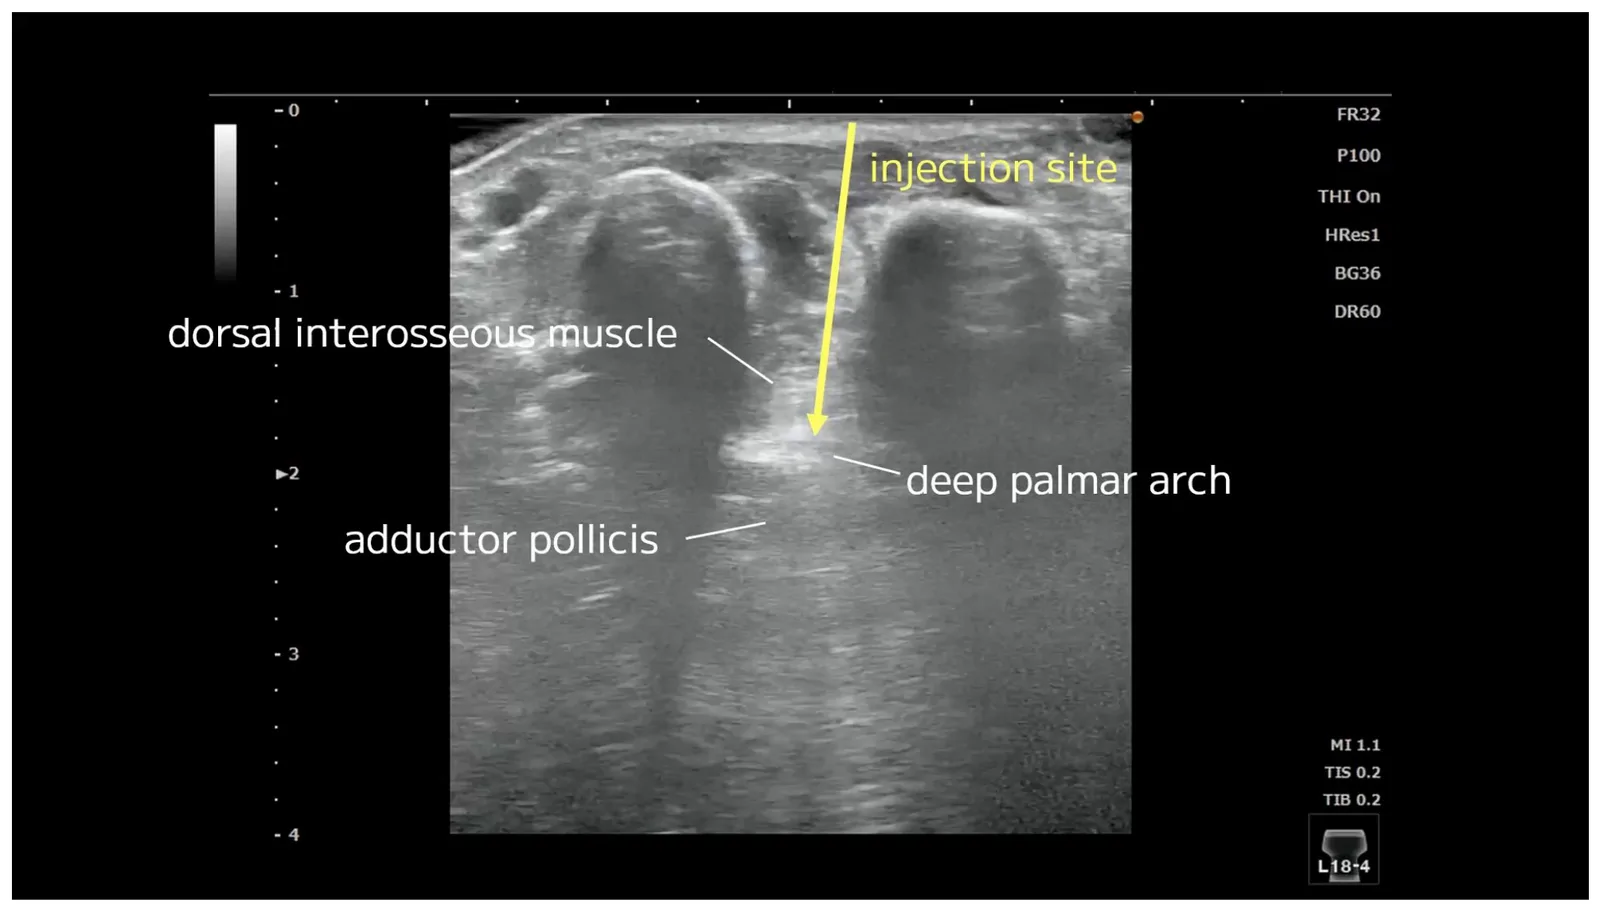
Point 9—deep palmar arch/dorsal and palmar interossei: ultrasound-guided fascia hydrorelease; yellow arrow indicates the injection site.
